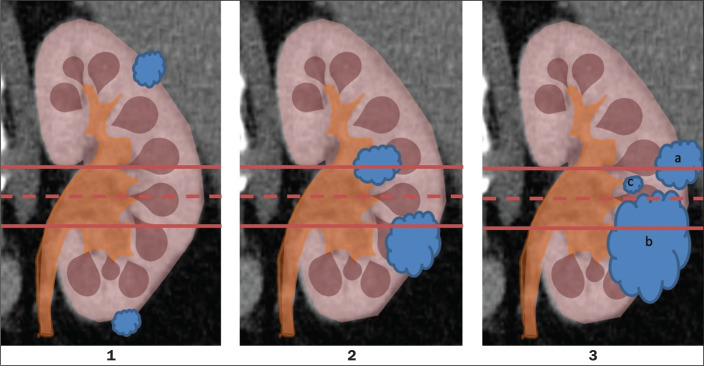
RENAL nephrometry score based on a CT scan. The numbers 1 to 3 represent points assigned to each category of tumor. The polar lines (solid lines) are determined in a sagittal view of the kidney. a, > 50% of the mass is above the upper polar line or below the lower polar line; b, the mass crosses the axial renal midline; c, the mass is entirely between the polar lines.
